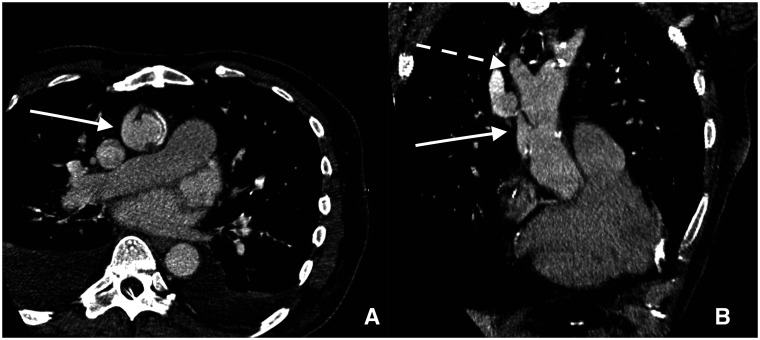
Preoperative imaging. (A, B) Multiplanar computed tomography angiography reconstruction in which the pseudoaneurysm at the distal anastomotic site (white arrow) and the brachiocephalic trunk origin (white dashed arrow) are clearly visible.
